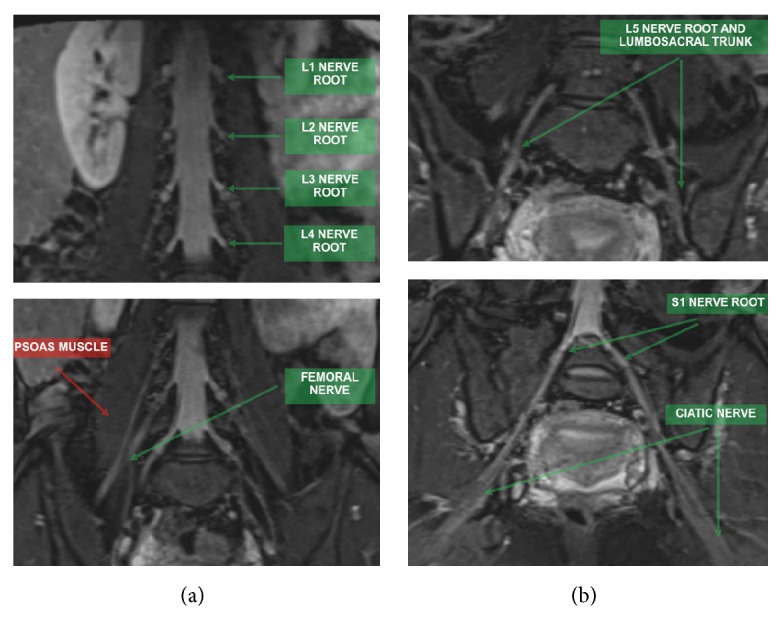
MR images in coronal STIR 3D SPACE (MIP) in (a), showing nerve roots of the lumbar plexus (L1 to L4) and the femoral nerve, formed by posterior nerve roots from L2 to L4, emerging lateral to the psoas muscle. In (b), MR images show L5 and S1 nerve roots, the lumbosacral trunk formed by the anastomotic branches of L4 and L5 nerve roots, and the sciatic nerve formed by major branches of the sacral plexus from L4 to S3.
